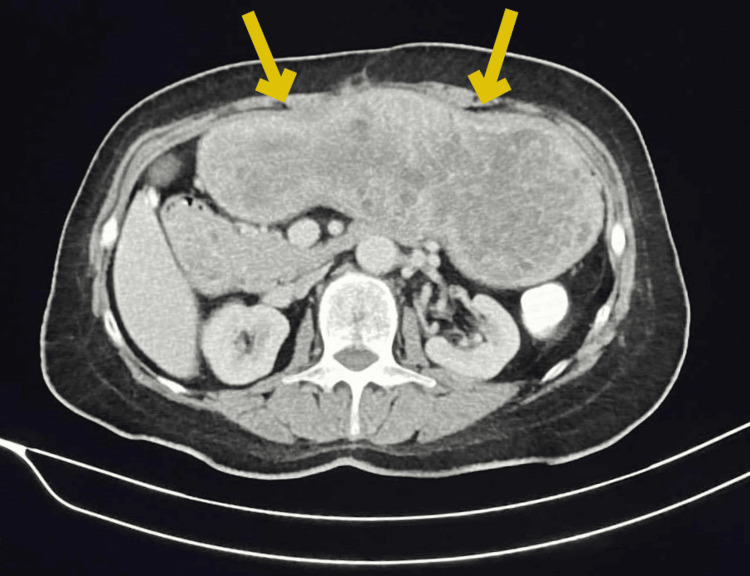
Abdominal CT scan of the distended stomach
